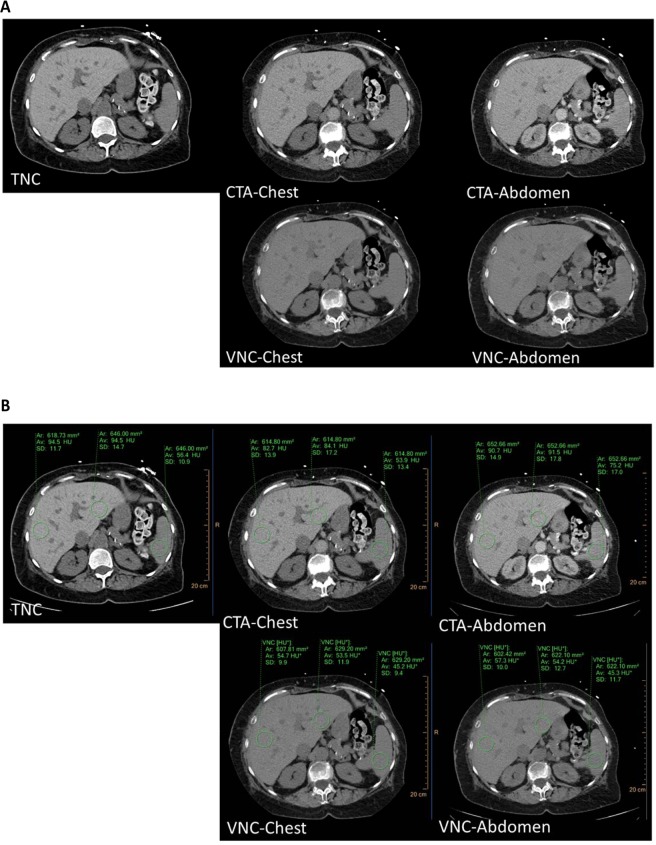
(A) Axial CT images in soft tissue window settings of the upper abdomen in a 72-year-old female receiving TAVR planning examination. The patient was treated with amiodarone 200 mg/day for nine months prior to imaging. The examination was conducted in February 2018. Depicted are true non-contrast (TNC), CT angiography (CTA) of the chest and abdomen (CTA-Chest and -Abdomen), as well as virtual non-contrast (VNC) from CTA-Chest (VNC-Chest) and CTA-Abdomen (VNC-Abdomen). Attenuation of the liver is increased in TNC (~95 HU) which is likely to be caused by amiodarone treatment and accumulation in the liver. Liver attenuation is noticeably lower in VNC-Chest and -Abdomen (~54–57 HU) compared to TNC (~95 HU) indicating that VNC not only subtracts iodine from contrast media but might also be able to subtract iodine from amiodarone deposits. However, attenuation in the spleen (where amiodarone usually does not accumulate) is comparable between VNC Chest- and -Abdomen (~45 HU) and TNC (~56 HU). (B) Images of Fig. 3A with additional ROI-placement.
